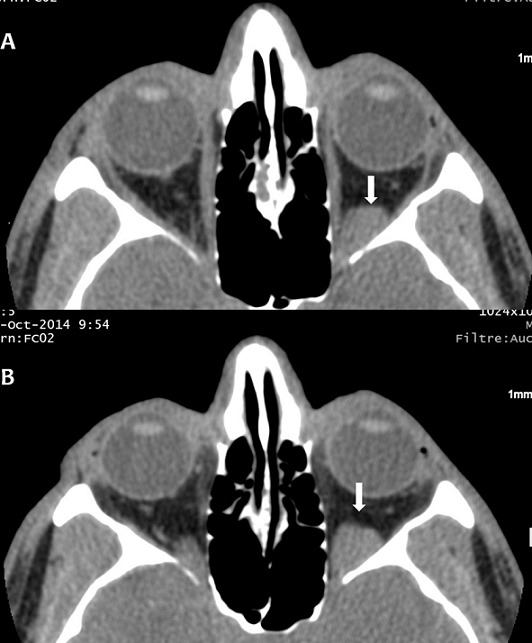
orbital CT in spontaneous contrast showing a left retro-orbital mass (A, arrow); note the volume increase of the mass in prone position (B, arrow)
